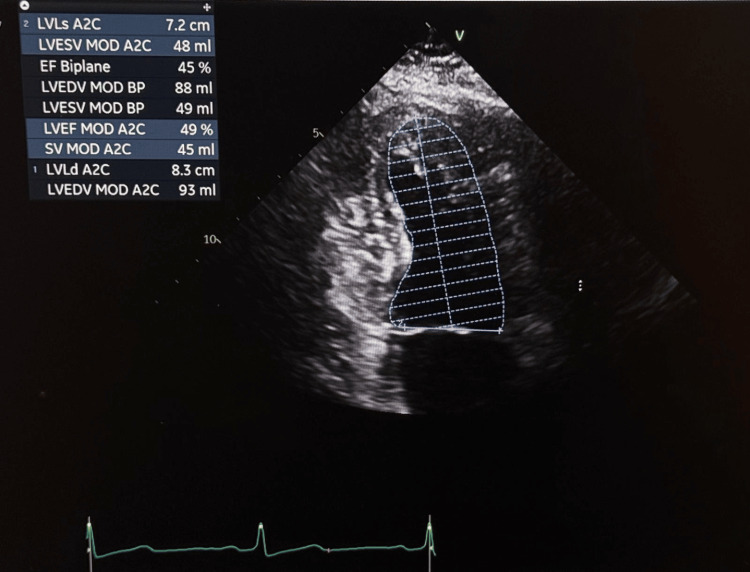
Transthoracic echocardiography demonstrating mildly impaired left ventricular systolic function with a biplane left ventricular ejection fraction of 45% calculated using Simpson’s biplane method.
